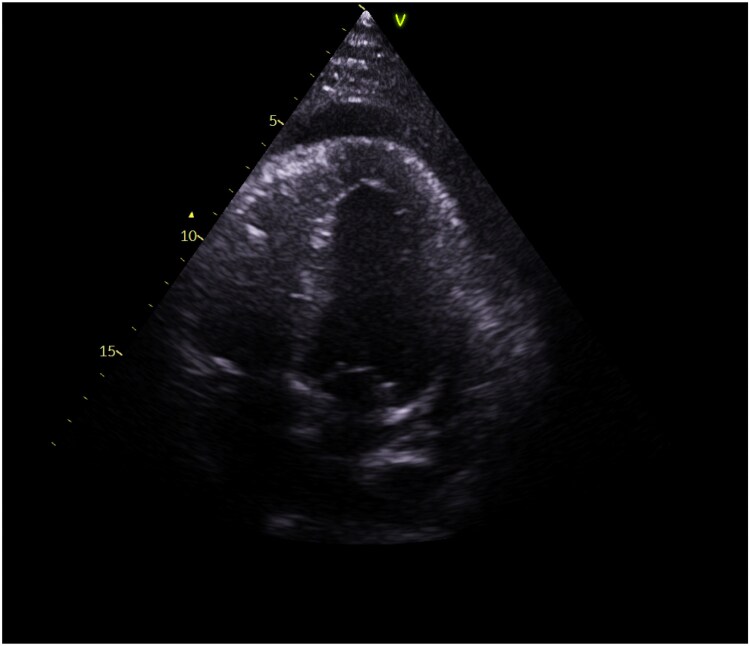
Echocardiogram pre-pericardiocentesis.
